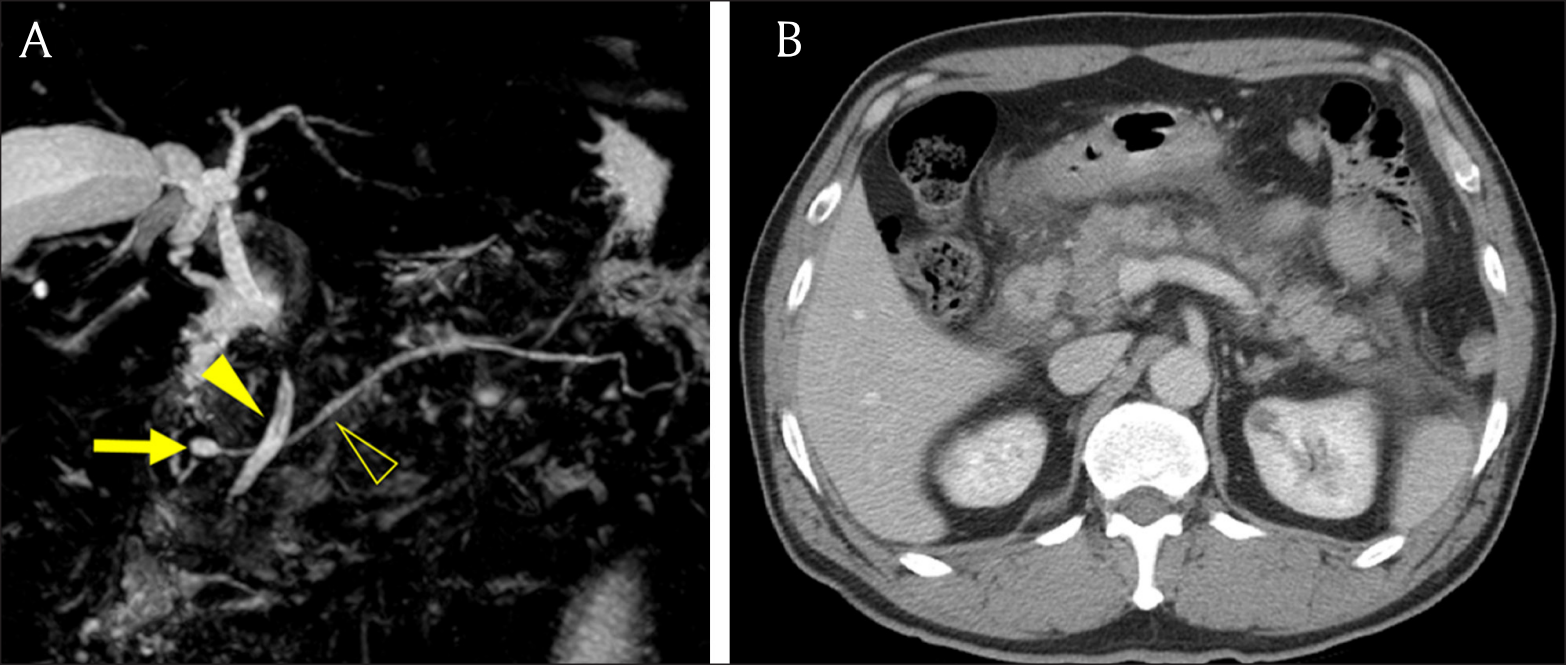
A 55-year-old man with pancreas divisum and Santorinicele who had history of recurrent pancreatitis. (A) MRCP image shows focal cystic dilatation of the distal portion of Santorini duct (arrow). Note crossed common bile duct (arrowhead) and main pancreatic duct (open arrowhead). (B) Axial portal venous phase CT image shows fluid collection around the pancreas, consistent with acute pancreatitis.
